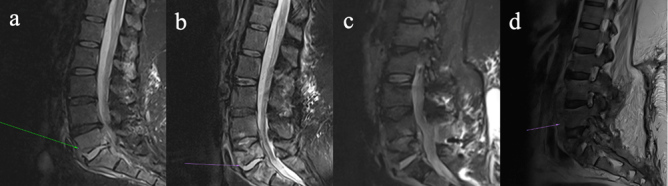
Four-panel figure demonstrating progressive discitis/osteomyelitis involving the lower lumbar spine on magnetic resonance imaging (a–d). (a) Sagittal STIR images show discitis/osteomyelitis at L5/S1. (b) Sagittal STIR images now demonstrate progression of disease, with erosion of the L5 superior endplate, development of an anterior epidural collection, and associated ligamentum hypertrophy with resultant bunching of the distal cauda equina. (c) STIR image pre-instrumentation removal demonstrate a progressive discitis/osteomyelitis at L4/L5, with multiple enhancing collections within the anterior epidural space and posterior to the anterior longitudinal ligament. (d) Sagittal T2 images of the lumbar spine post-hardware removal demonstrate further destruction of the L5 vertebral body, with a persistent enhancing collection within the anterior epidural space, and a new collection along the anterior longitudinal ligament.
